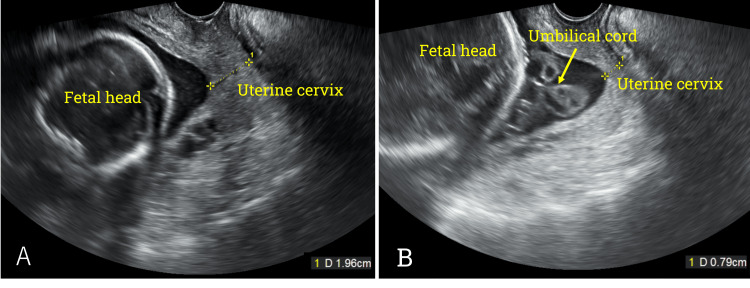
Transvaginal ultrasound findings A: At 26 weeks gestation, the cervical length was 1.96 cm. B: At 33 weeks gestation, a forelying umbilical cord was revealed between the fetal head and the uterine cervix (yellow arrow). The cervical length was shortened to 0.79 cm.
